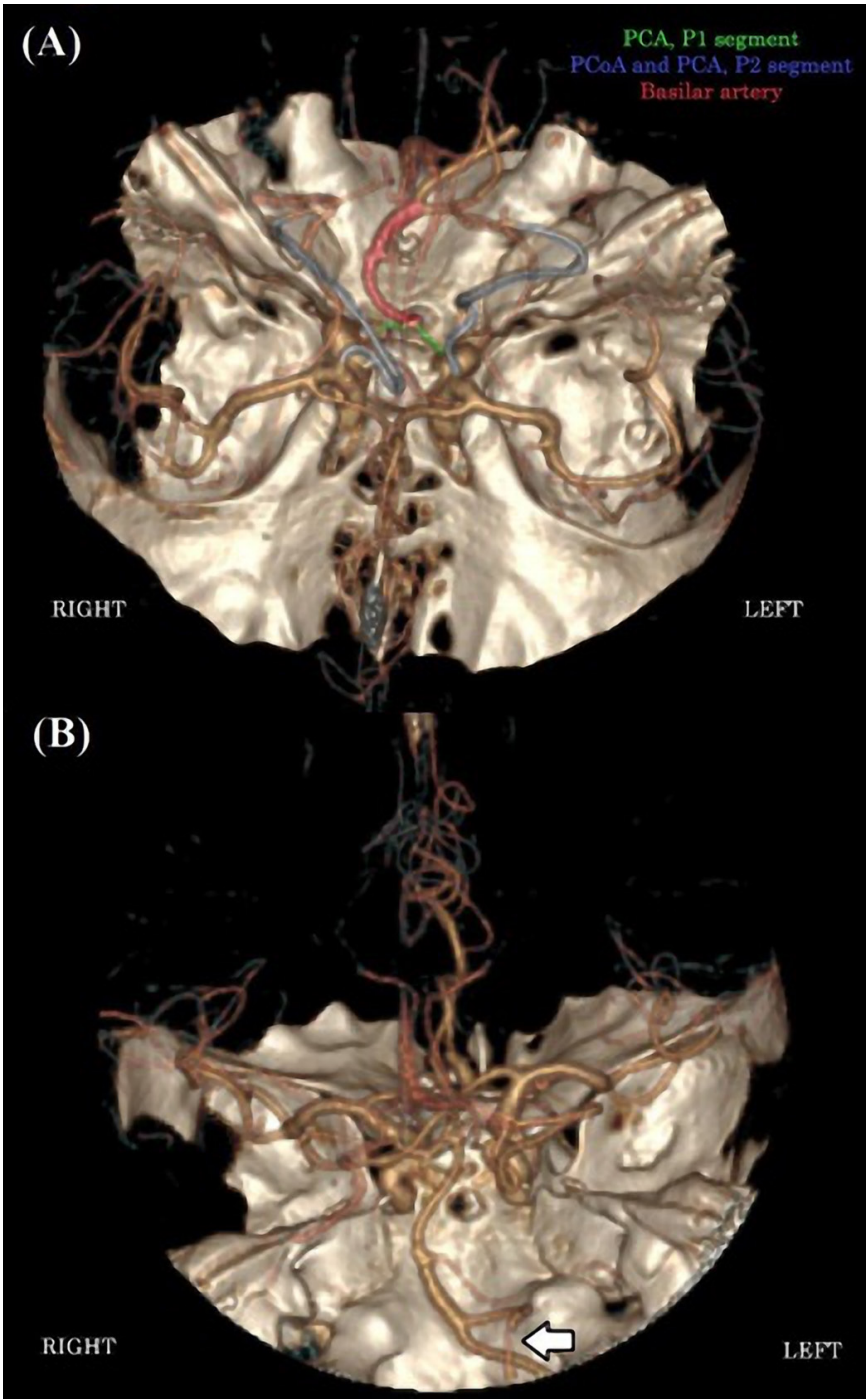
CT Angiography. Posterior circulation revealing both P1 segments of the posterior cerebral arteries are abnormally narrow (A). Arrow pointing to a tubular stenosis of the V4 segment of the left vertebral artery (B). PCA – Posterior cerebral artery; PCoA – Posterior communicating artery.
